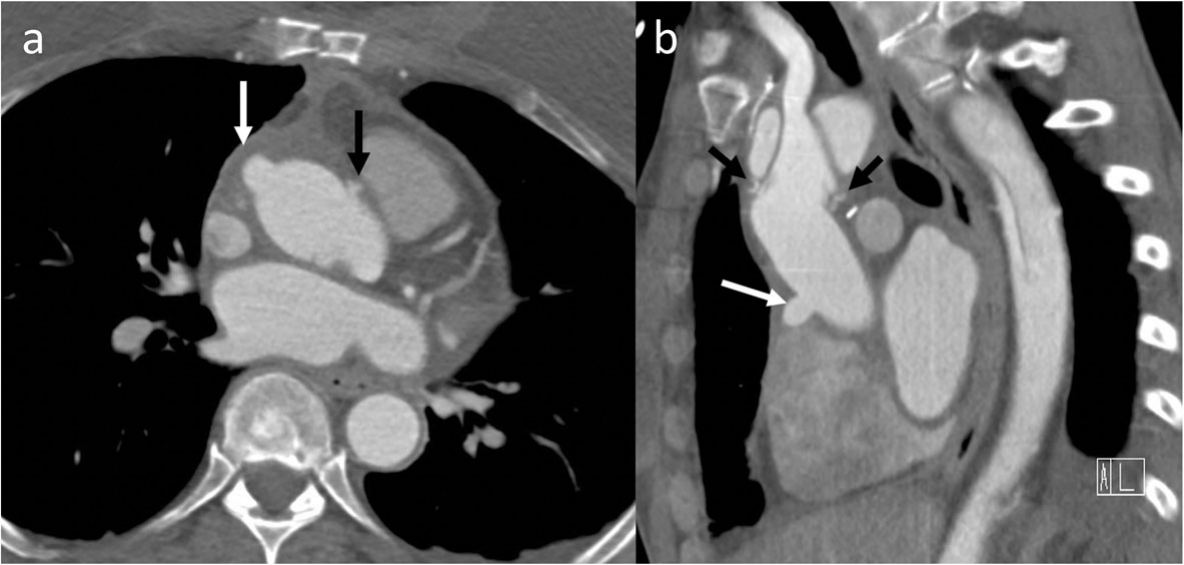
A 58-year-old female post-op day 10 status post supracoronary ascending aorta replacement with graft repair and concern of pseudoaneurysm on a pulmonary embolism rule-out CT (not shown). Axial (a) and sagittal (b) high-pitch CTA images show a smooth contrast filled outpouching from proximal graft (white arrow) near to the anastomosis (black arrow in a). This smooth outpouching is a normal post-operative appearance, related to over sewn reperfusion catheter stump from cardiopulmonary bypass during surgery
